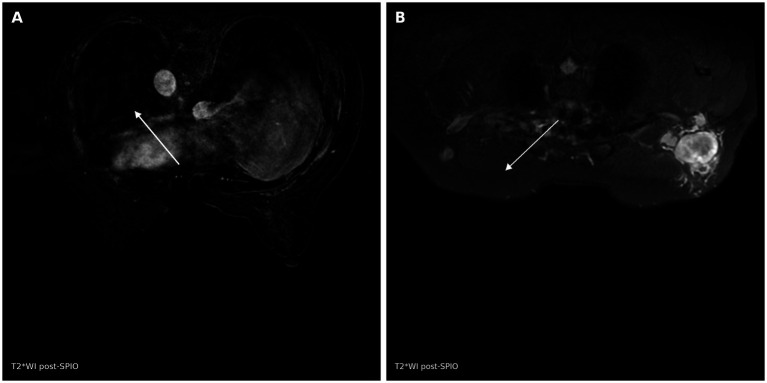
Representative SPIO-enhanced MRI appearances of lymph nodes. (A) Post-SPIO T2*-weighted image of a reactive hyperplastic axillary lymph node (arrow) demonstrating marked signal loss, consistent with macrophage-mediated iron uptake. (B) Post-SPIO T2*-weighted image of a metastatic axillary lymph node (arrow) showing preserved signal intensity, consistent with reduced iron uptake due to tumor cell replacement of normal lymphoid tissue. Field strength: 3.0 T; imaging performed 12 h after intravenous administration of SPIO (Resovist, 0.2 mL/kg).
